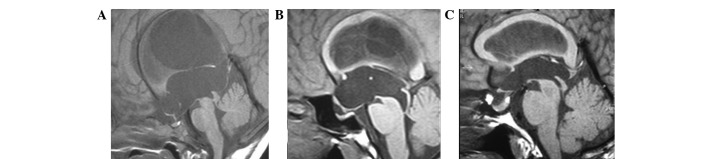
Mid-sagittal magnetic resonance imaging (MRI) revealed a membranous septum and the third ventricular floor was flat. (A and B) The aqueduct membranous obstruction revealed the goblet-like change. (C) The aqueduct membranous obstruction without the goblet-like change.
